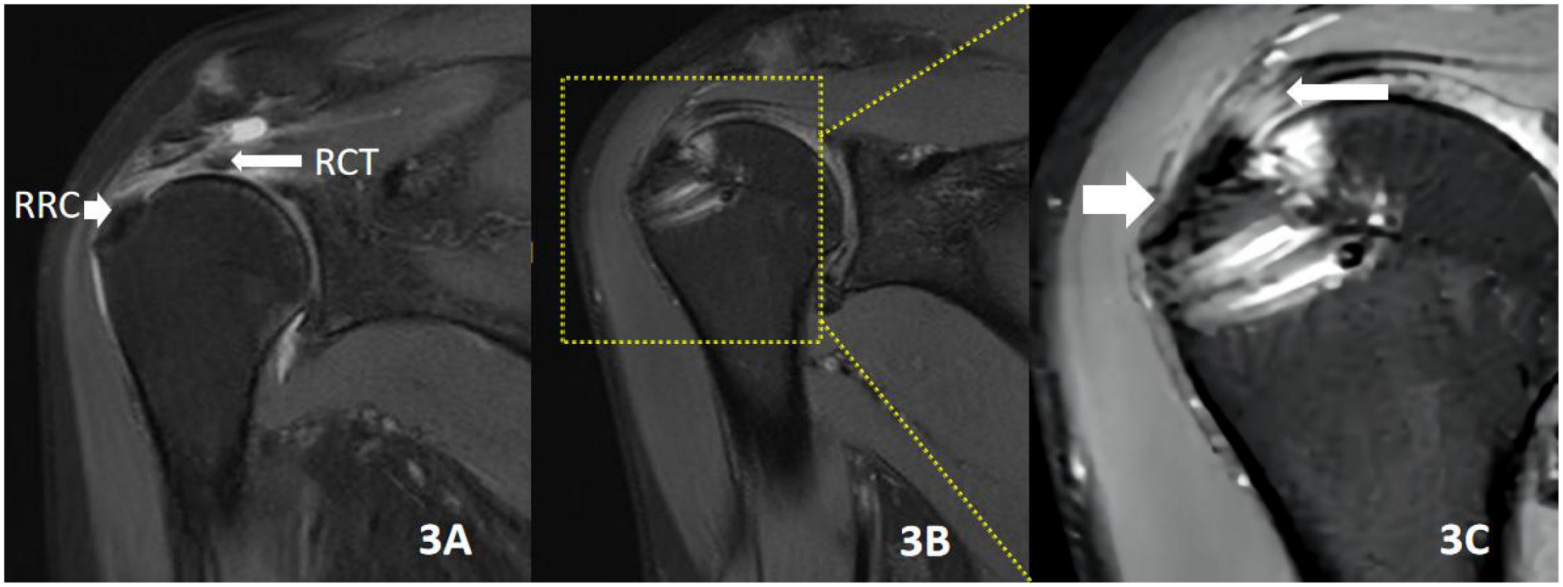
Male patient, 55 years old, traumatic injury causing shoulder pain and discomfort for 4 months, undergoing remnant-preserving techniques repair. (A) RCT (proximal rotator cuff tear), RRC (remnant rotator cuff in the footprint area). (B) MRI of the shoulder joint repeated 2 years after surgery, with good rotator cuff healing. (C) A magnified 3× image in the yellow box in B. The short white arrow on the left indicates the preservation of the original rotator cuff migration structure in the rotator cuff and footprint area after the remnant-preserving technique. The long white arrow on the right indicates the tendon–tendon healing formed in the torn portion of the rotator cuff.
